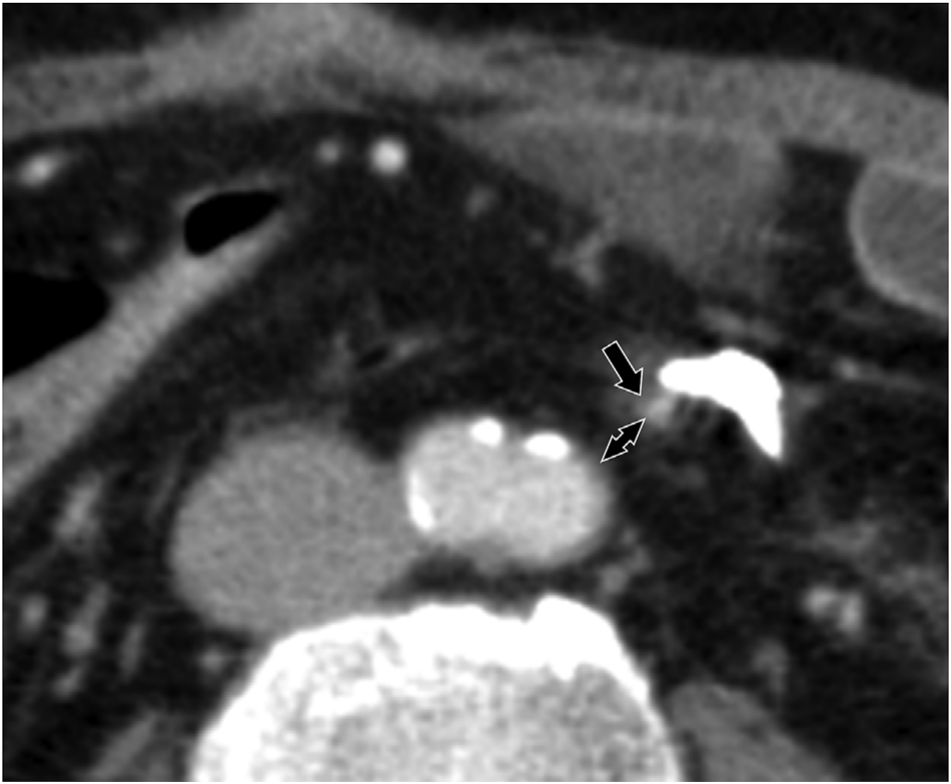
Distance between the superior rectal artery (SRA; arrow) and abdominal aorta at the aortic bifurcation level (double-headed arrow) was measured on computed tomography (CT) images obtained 3–6 months after SRA-preserving surgery.
